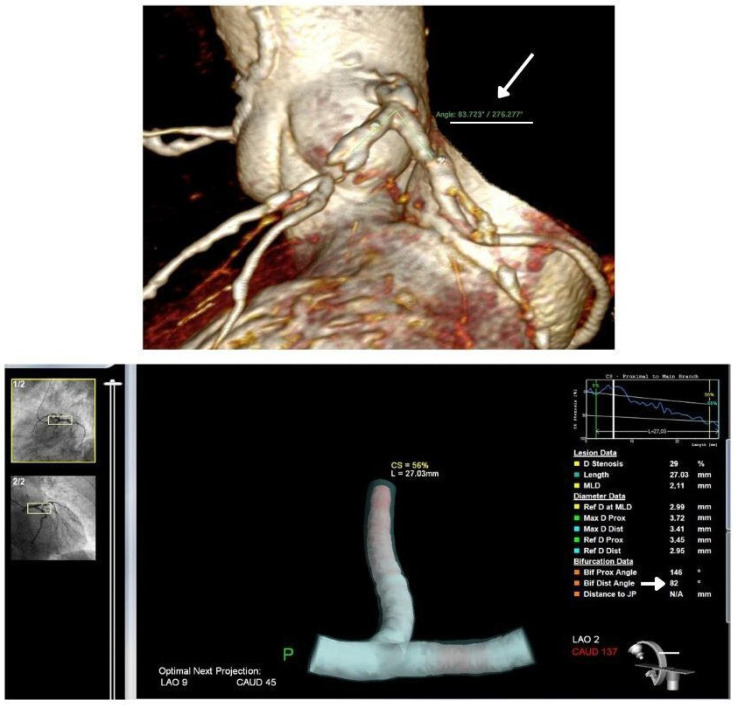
The left main bifurcation angle (LMBA) was measured on CTA (top panel) and on coronary angiographic images using 3D-QCA (bottom panel).
